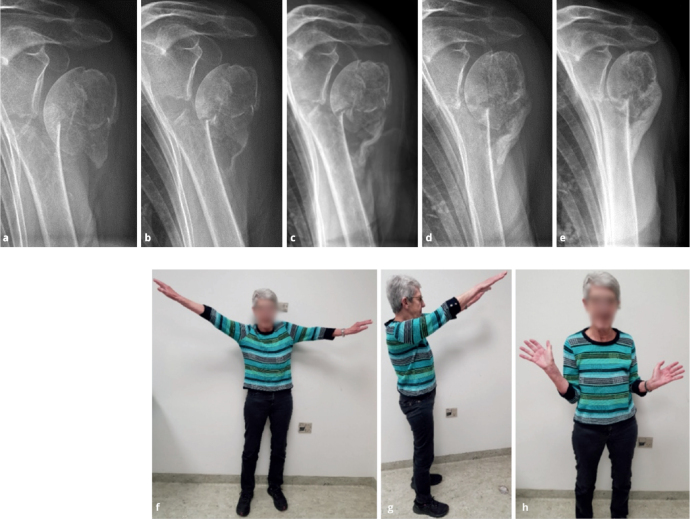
Left 2-part surgical neck fracture with medial translation of the humeral shaft in an 80-year-old female. Anterior–posterior radiographs at 2, 4, 6, 12, and 24 weeks (a–e). Photos (f–h) at 6 months after injury. At 24 weeks, OSS was 41 (84%), and EQ-5D-3L was 0.78.
